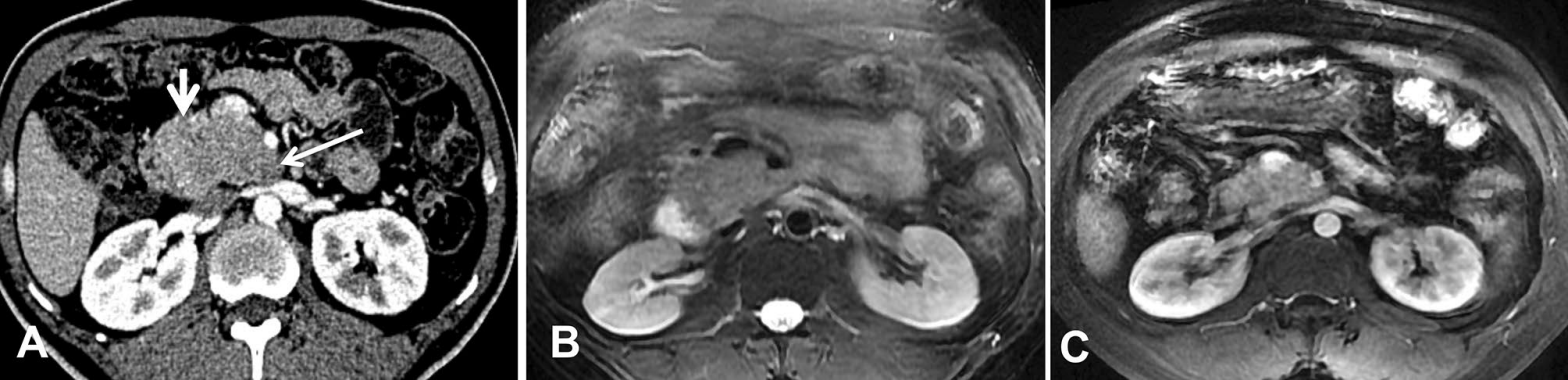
CT and MRI images of a 36-year-old man with pathologically confirmed PB. (A) In the AP view, the tumors presented as exogenous hypovascular masses in the head of the pancreas. The tumor exhibits an exophytic growth pattern, similar to branches growing outward from a tree trunk, and the long arrows indicate that the tumors have a tapering head. The short arrows indicate that the interface between the root of the tumors and the head of the pancreas is not clear. The MRI examination showed that the mass was isointense on T2WI (C).
